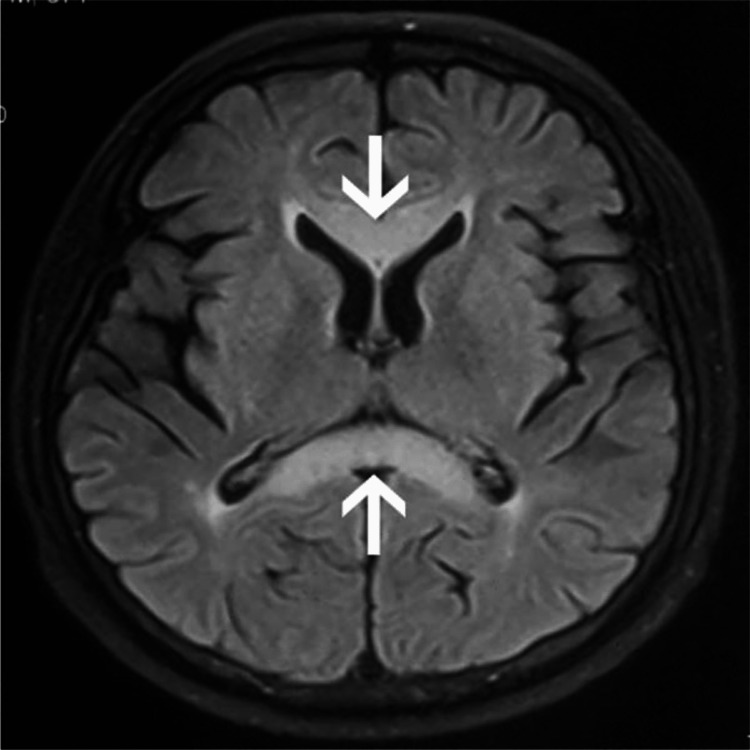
FLAIR showing abnormal hypointensity in corpus callosum Reference [30] is licensed under CC BY-NC 4.0
